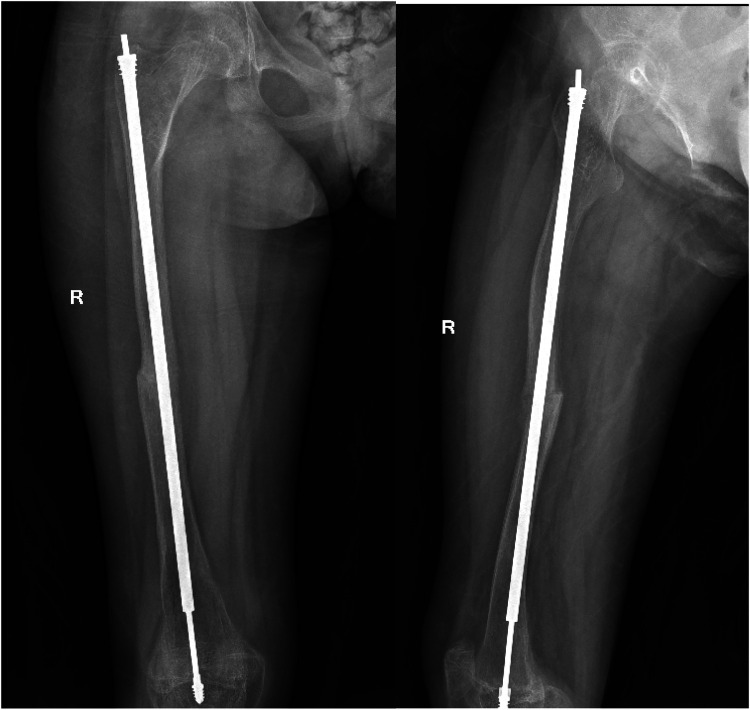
Repeat X-ray at 6 months postoperatively showing a well-healed fracture.
